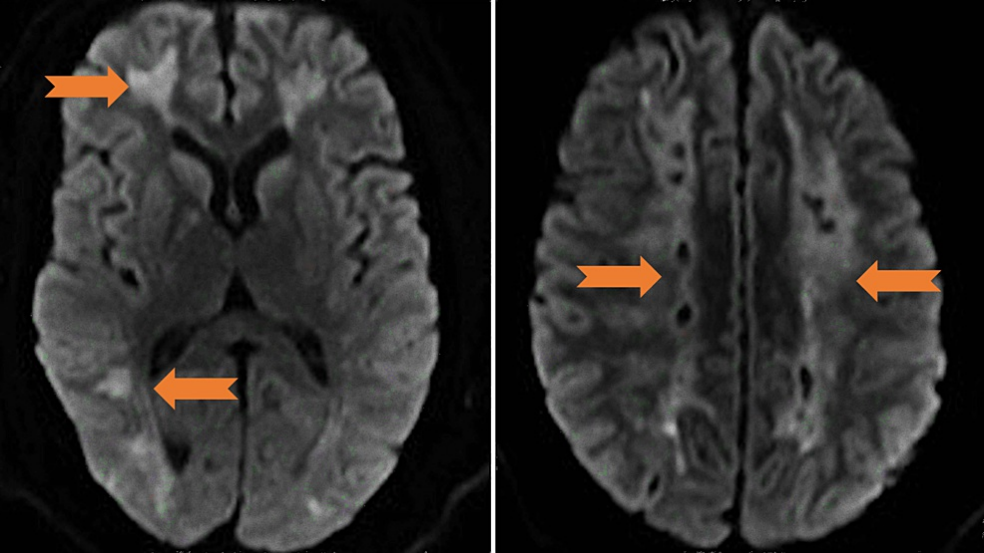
DWI sequence with mild restricted diffusion and patchy non-mass-like enhancement at bilateral subcortical regions without mass effect (arrows). DWI: diffusion-weighted imaging
